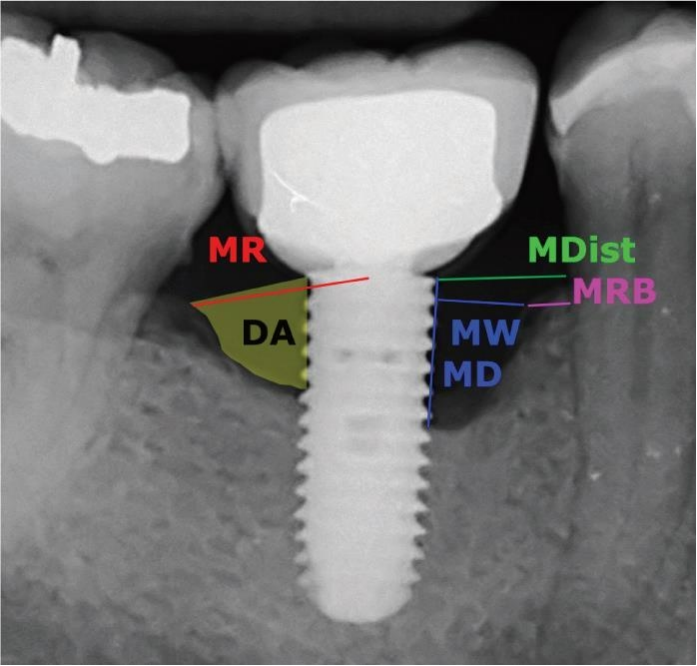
Graphic representation showing the radiographic measurements (MR: maximum radius; MDist: mesial distance; MD: mesial depth; MW: mesial width; MRB: residual mesial bone; and DA: distal area).
